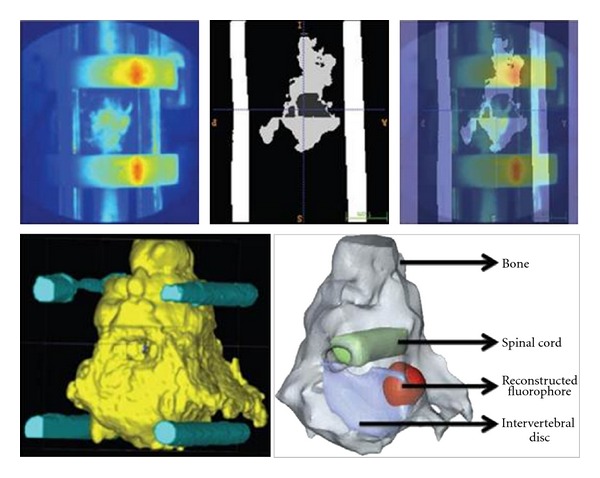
3D visualization of the reconstructed fluorophore. The disc (blue region) and the spinal cord (green region) are provided but obtained from the MRI segmented model.
